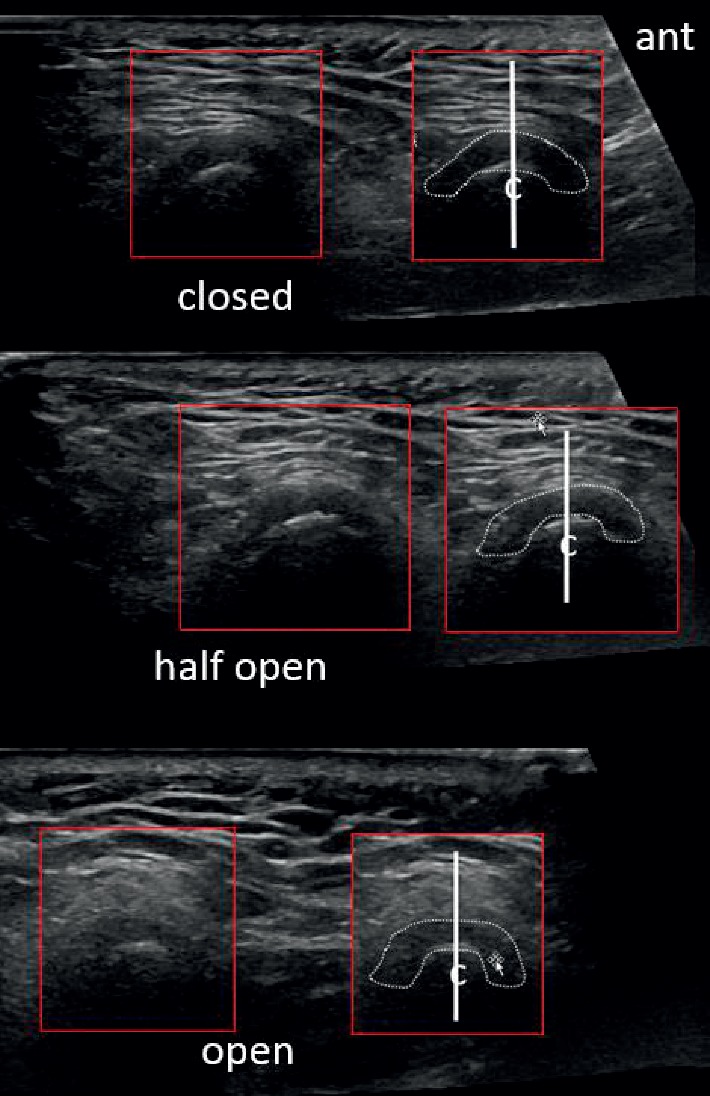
Normal ultrasound appearance of the articular disc in the sagittal plane, seen as an inverted hypoechoic c-shaped structure. Note that the disc maintains a constant central appearance with respect to the centre of the condyle, “c,” outlined by a central vertical line during the closed-mouth, half-open-mouth, and fully-open-mouth views. The anterior (“ant”) band and posterior band of the articular disc appear symmetrical in size with respect to the centre of the condyle. A focal annotated view was provided to aid visualization with the articular disc outlined with a dotted contour.
